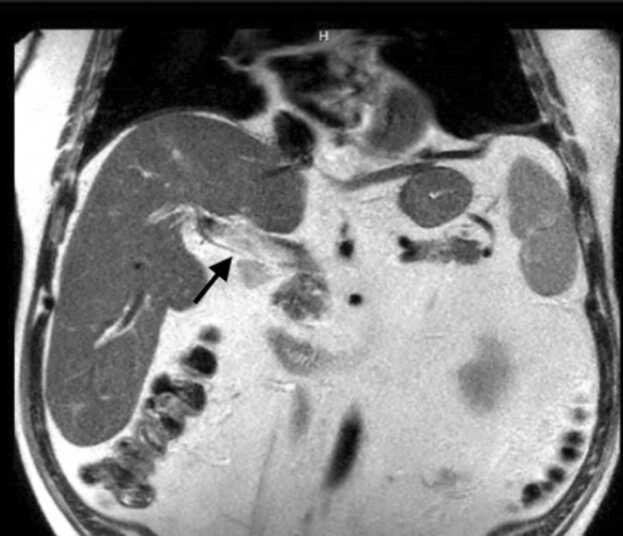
MRI of the abdomen and pelvis without contrast showing evidence of thrombus portal vein (black arrow).
